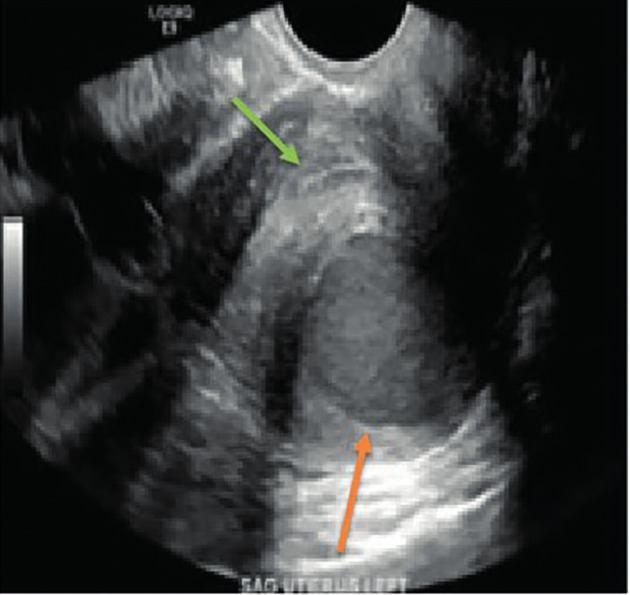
2D transvaginal ultrasound picture of a juvenile cystic adenomyosis in the posterior wall of the uterus (orange arrow). The picture is showing the endometrial strip separate from the cyst (green arrow).
